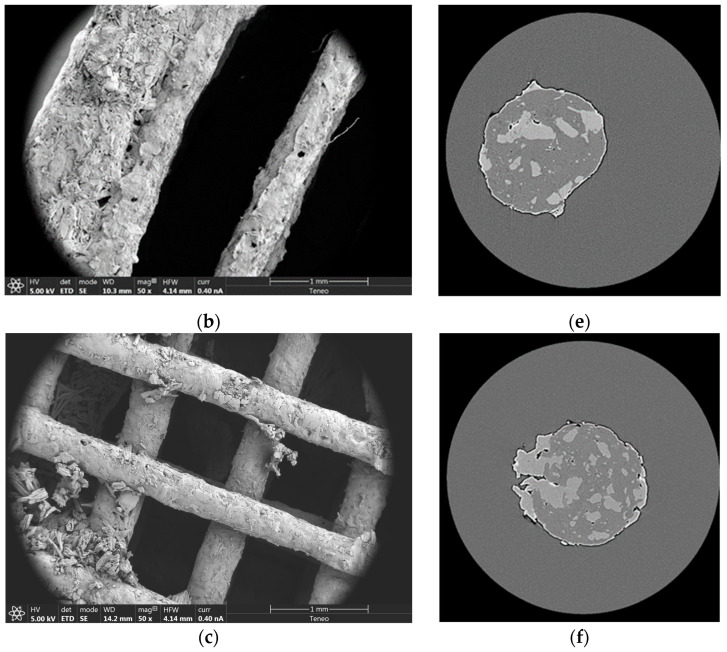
SEM images at 50X of the inner structure of the systems printed with 20% (a), 30% (b), and 50% (c) drug loading, and images made with X-ray tomography of the cross-section of the strands of the same drug percentages (20% (d), 30% (e), and 50% (f)).
